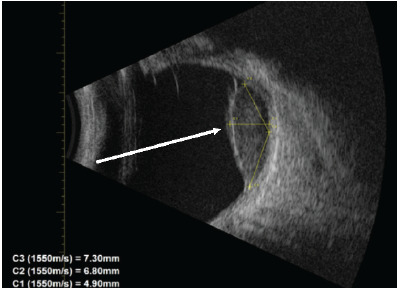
B-scan ultrasound of the left eye demonstrating serous sub-retinal fluid and choroidal mass (arrow).
